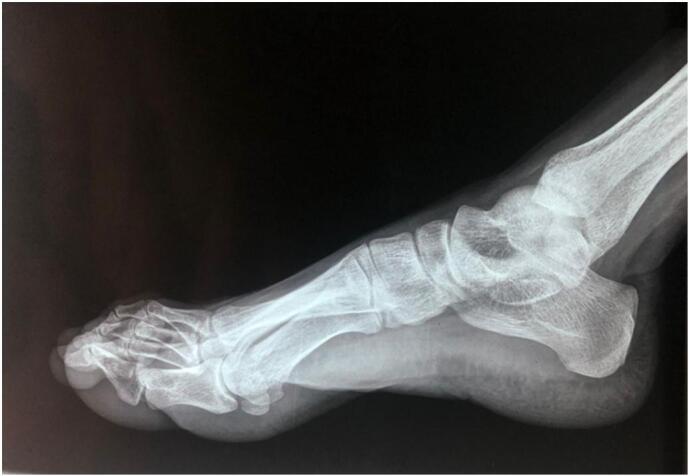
Standard foot X-rays showing soft tissue thickening without visible calcifications, bone lesions or osteolytic images.
